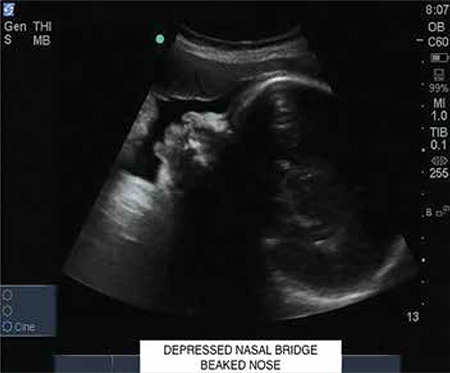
Ultrasound image (2-dimensional) of fetal facial profile showing mildly depressed nasal bridge with a beaked nose
